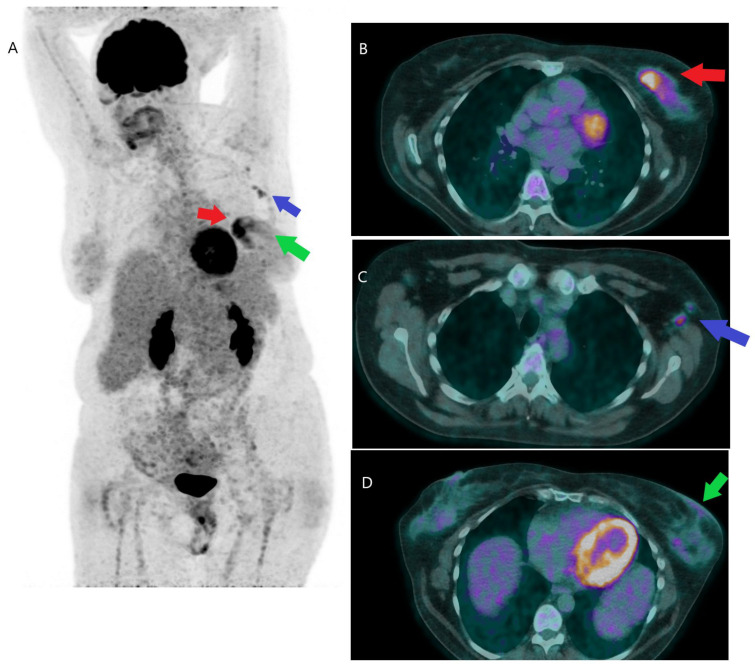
FDG PET/CT in initial staging. A 52-year-old woman with a newly diagnosed inflammatory left breast invasive ductal carcinoma of 29 mm, was referred for initial staging with FDGPET/CT. The PET/CT MIP image (A) shows the primary cancer (red arrow), axillary adenopathies (violet arrow), and cutaneous involvement (green arrow). Image (B) (fusion image, trans-axial view) shows the primary lesion of the left breast (red arrow). Image (C) (fusion image, trans-axial view) shows the axillary lymph node involvement of Berg’s level I (violet arrow). Image (D) (fusion image, trans-axial view) shows the cutaneous involvement (green arrow).
